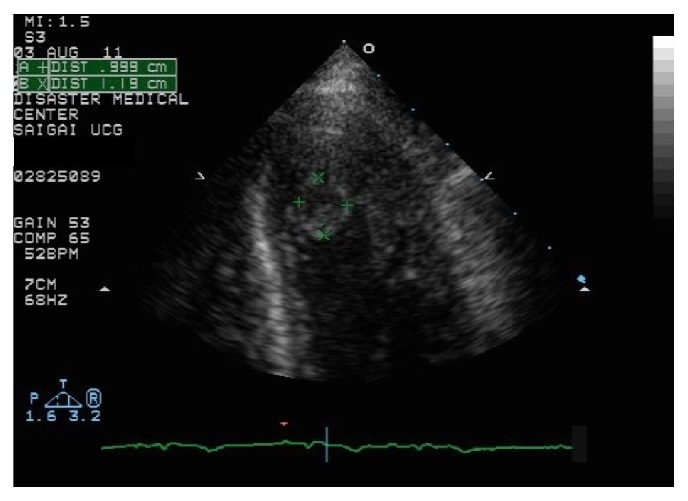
Echocardiogram showed a mobile mural thrombus in the apex of left ventricle.
